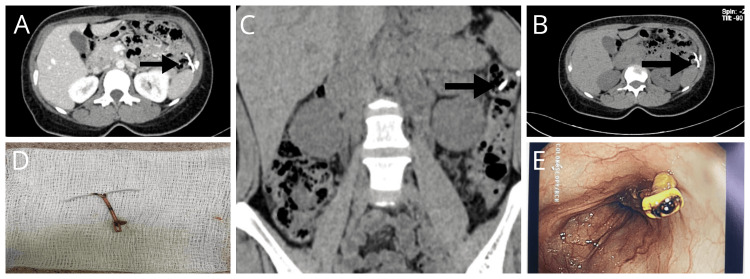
CT of the abdomen showing the IUCD in the descending colon (A, B, and C), the IUCD removed (D), and hemostasis secured endoscopically (E) CT: computed tomography, IUCD: intrauterine contraceptive device
